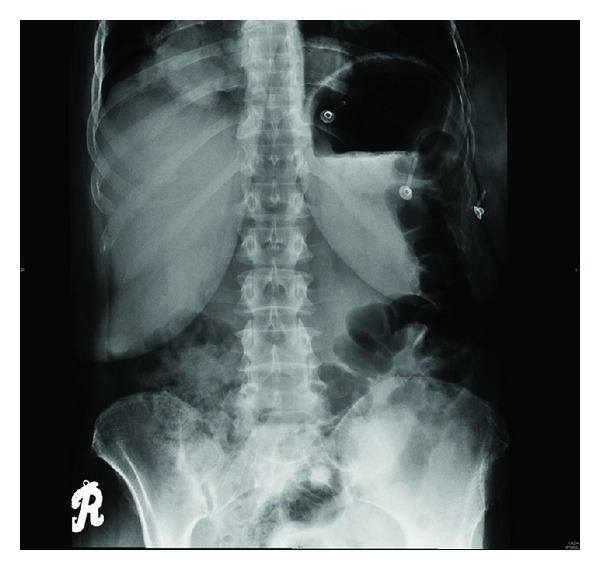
Plain abdominal radiograph showing dilatation of small bowel without any gas-fluid levels.
